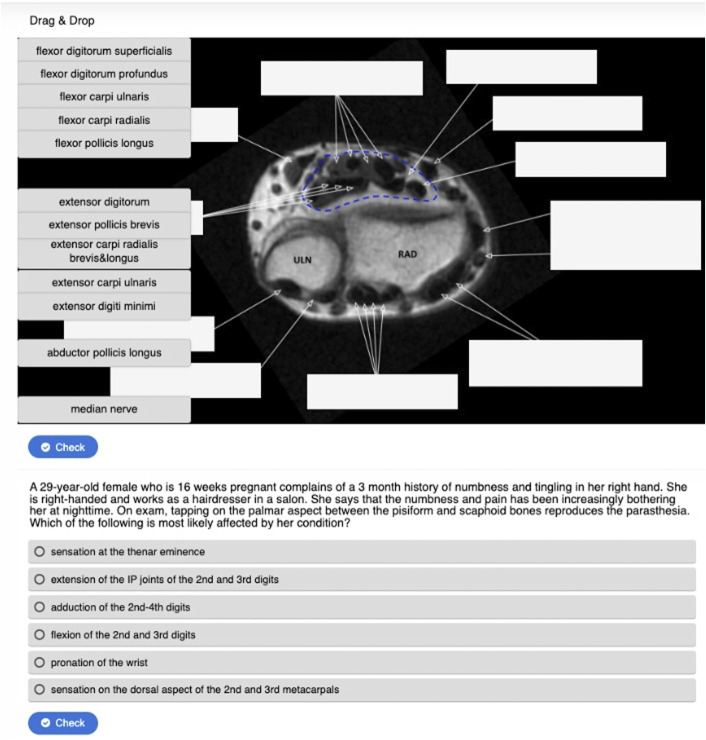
Example of an interactive recall exercise with MRI anatomy and a related multiple-choice question within the upper limb module. MRI, magnetic resonance imaging.
